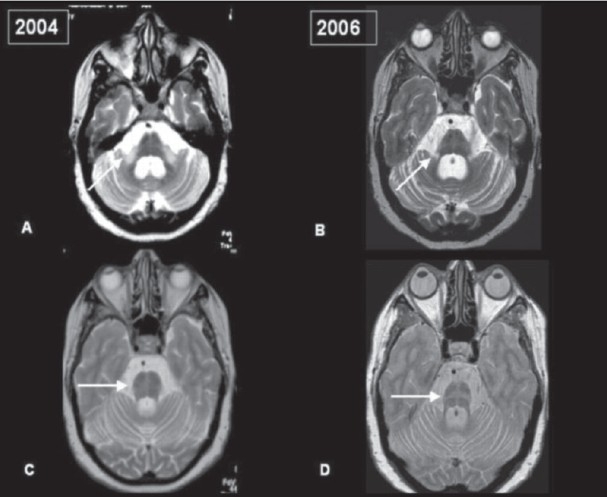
Serial MRI of brain of a patient with MSA-c over a 2-year period. A, B: T2W axial sequences showing hyperintensity of bilateral middle cerebellar peduncle. The repeat image after two years (B) revealed mild increase of brainstem and cerebellar atrophy and decrease in signal change. ‘Hot cross bun’ sign is also evident. C, D: PD axial images revealing the characteristic ‘hot cross bun’ sign in pons (2004) and after two years (2006)
